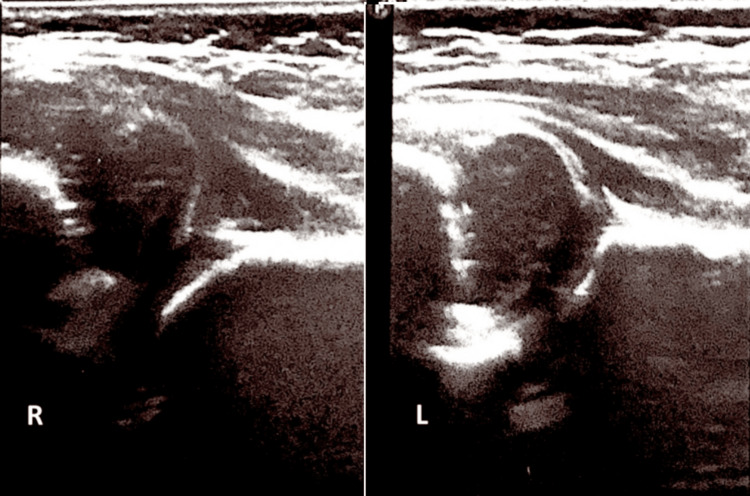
Ultrasound image demonstrating right hip dislocation in the developmental dysplasia of the hip. R: right. L: left
